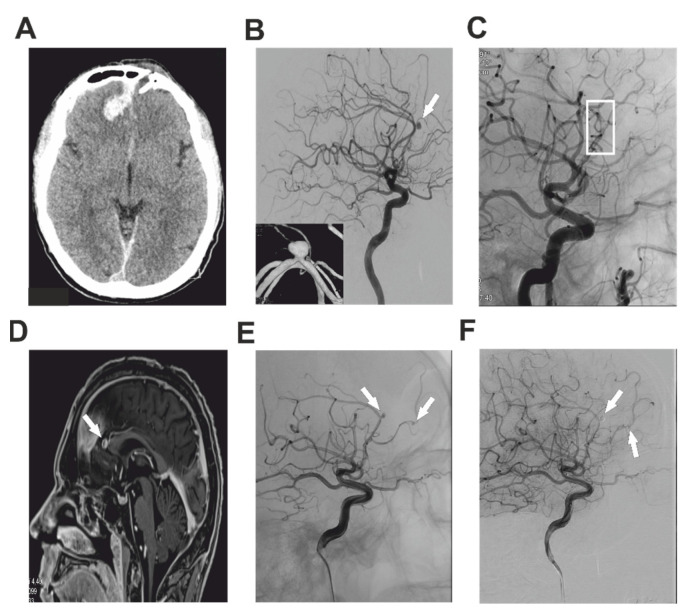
Imaging studies describing Case 1. Post-traumatic preoperative CT of the head showing intracerebral hematoma of the right frontal lobe with bone defect of the left side (A). DSA investigation with the white arrow pointing at the tIPA of the callosomarginal artery (B). DSA after treatment of the pseudoaneurysm using stent implantation (white box) (C). White arrow pointing on the growing remnant of the aneurysm of dACA several weeks after the primary treatment (D). Repeated DSA investigation. White arrowsshowing the growing partially-treated callosomarginal tIPA together with newly formed tIPA of the frontopolar artery on the right side (E). After successful embolization of both tIPAs, control DSA showed no residual flush in the tIPAs (white arrows) (F).
